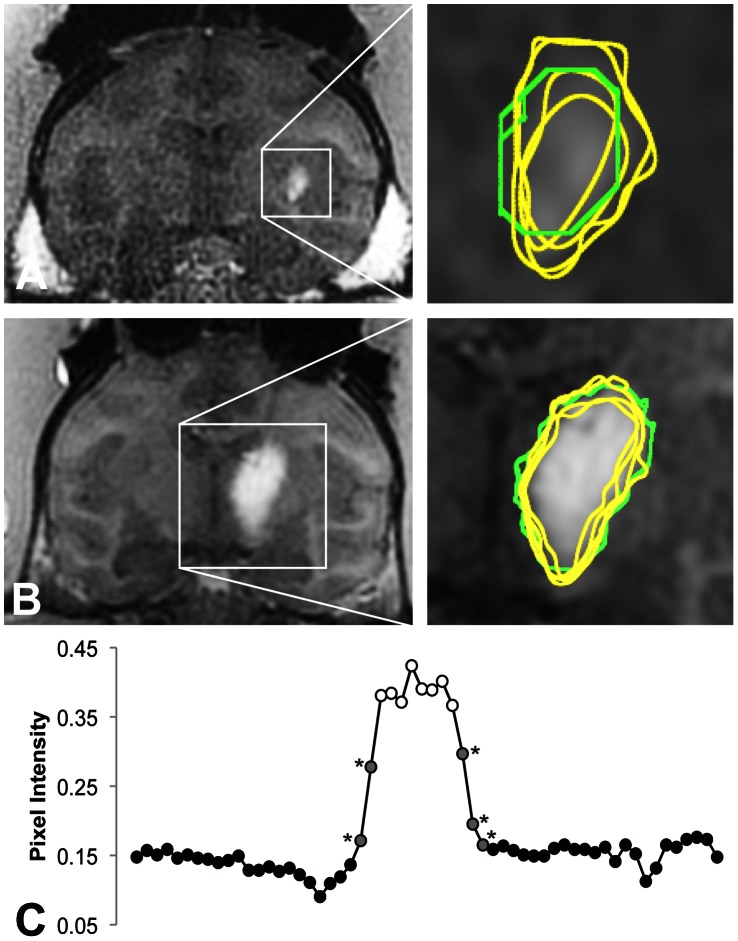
Representative manual (yellow) and automated (green) segmentations of the T1-weighted FLASH images. The automated segmentations were more consistent in both a (A) a 50 µL infusion in the putamen (manual: 177/226/131/232 µL; automated: 156/154/161/154 µL) and (B) a 160 µL infusion in the thalamus (manual: 425/505/364/564 µl; automated: 402/454/522/497 µL). (C) A cross-section of the thalamus infusion along the dotted line demonstrated that the inconsistently classified pixels (gray, starred) were on the shoulder of the infusion.
